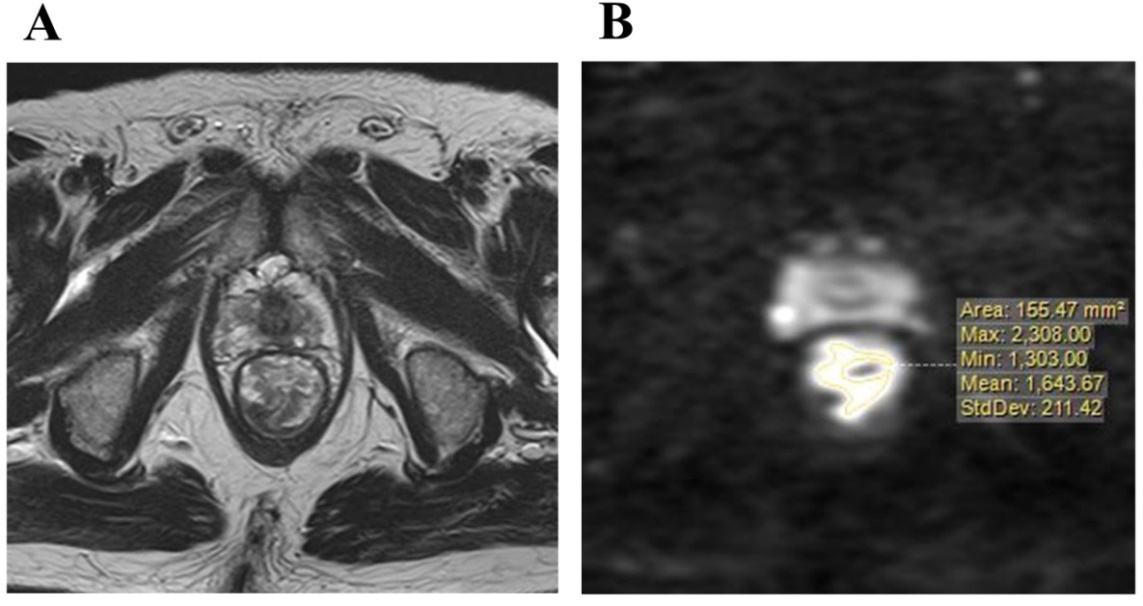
A: T2-weighted axial image of the maximum cut surface of lower rectal carcinoma, B: The distribution signal intensity on a diffusion-weighted image in the maximum cut surface was measured.
